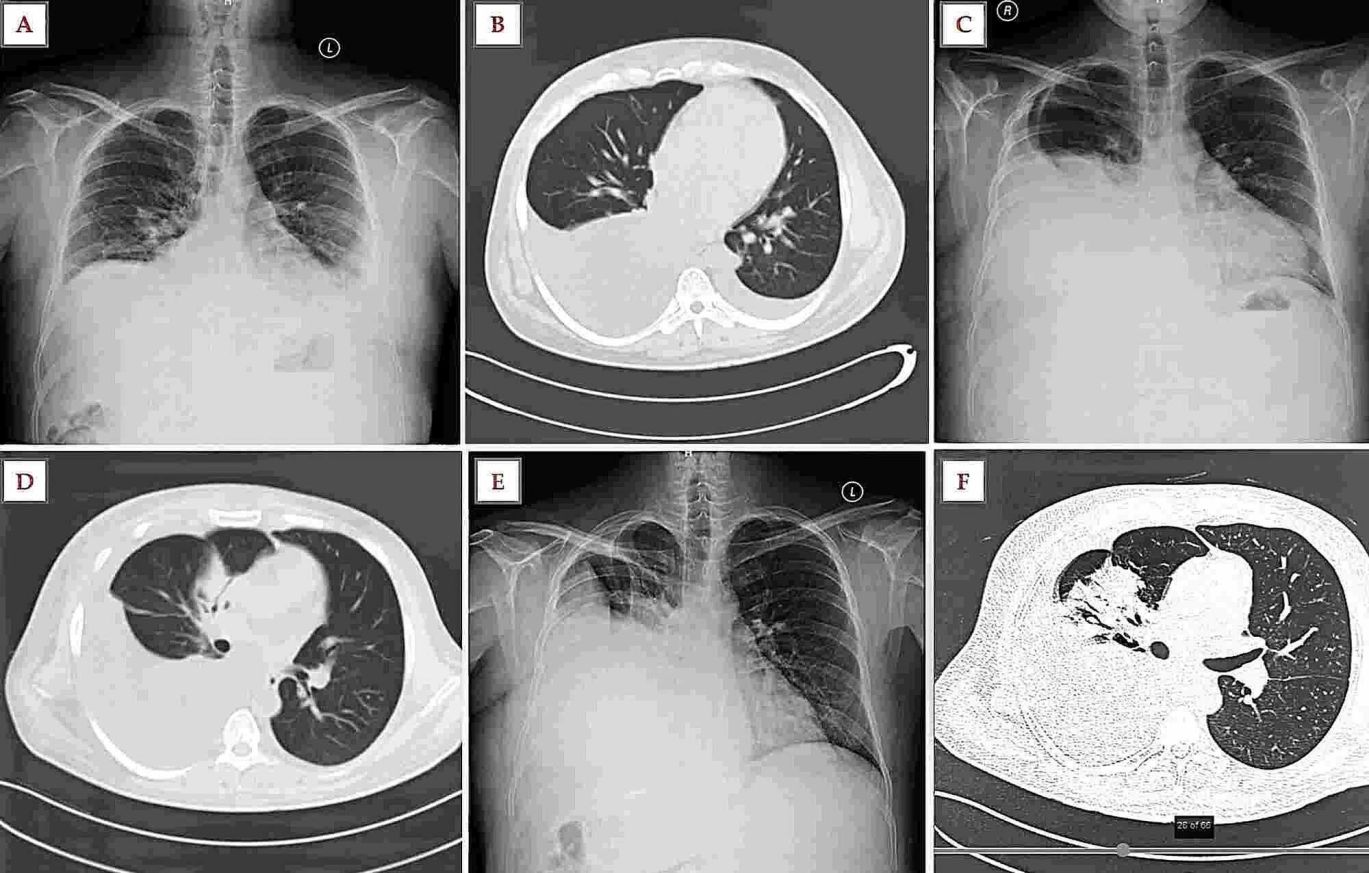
Imaging during the first two admissions. (A) Chest radiography (following thoracocentesis) during the previous admission shows mild left-sided pleural effusion and right-sided costophrenic angle blunting. (B) CT chest during the previous admission revealing bilateral effusion (R>L). (C and D) Chest radiography and CT chest at admission (current) showing right-sided pleural effusion. (E) Chest radiography on day nine shows right-sided moderate-to-submassive pleural effusion (following three sittings of therapeutic thoracocentesis). (F) CT chest on day nine reveals a right-sided pleural effusion and right middle lobe pneumonia. CT, computed tomography
